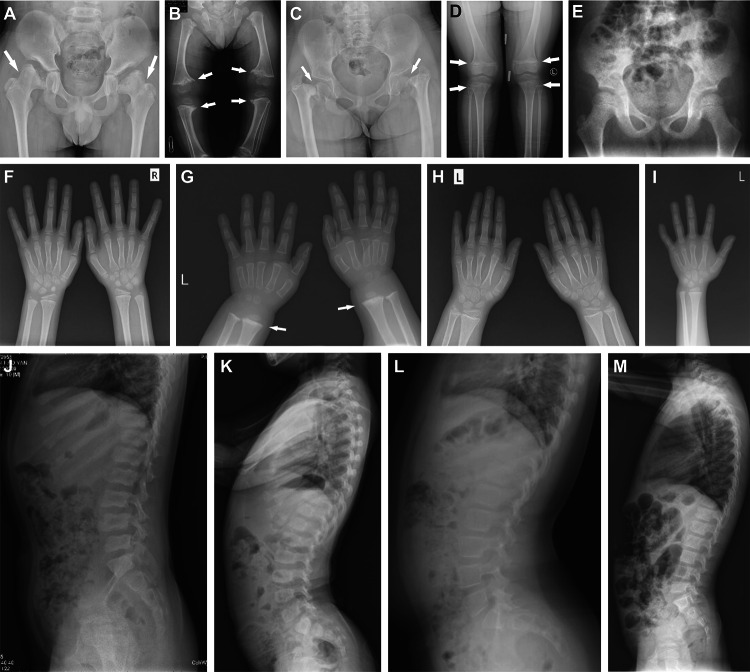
Radiographic features of 4 patients with MCDS in PUMCH. A, F, J Radiographs of pelvis, hand, and thoracolumbar spine of patient 1. The arrows indicate widening of the femoral epiphysis and a short femoral neck. The vertebrae were irregular and flat. The distal ulnar and radial epiphyses were normal. B, G, K Radiographs of the lower extremities, hand, and spine of patient 2. Arrows indicate metaphyseal widening of distal femurs and proximal tibiae, and brush changes of the distal ulnar and radial metaphyses. The spine showed lumbar lordosis and flat vertebrae. C, D, H, L Radiographs of pelvis, knee, hand, and thoracolumbar spine of patient 3. Arrows indicate coxa vara and a slightly enlarged epiphysis of the knee. Lumbar lordosis was obvious. The distal ulnar and radial epiphyses were normal. E, I, M Radiographs of pelvis, hand, and spine of patient 4. The femoral, distal ulnar, and radial epiphyses were normal. The vertebral pedicles were overlong
